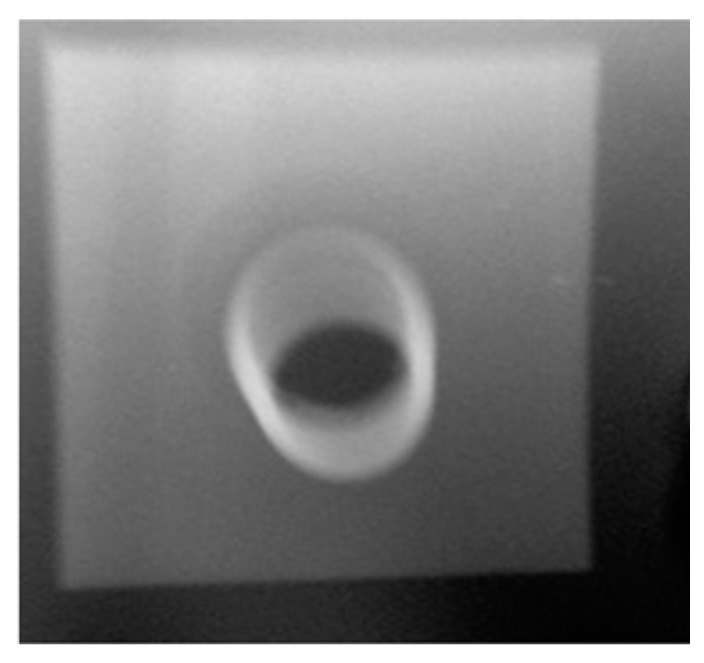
Radiography test sample FWTPET sample of rotational tool speed (1300 rpm), projection (1 mm), and depth of cut (0.5 mm).
